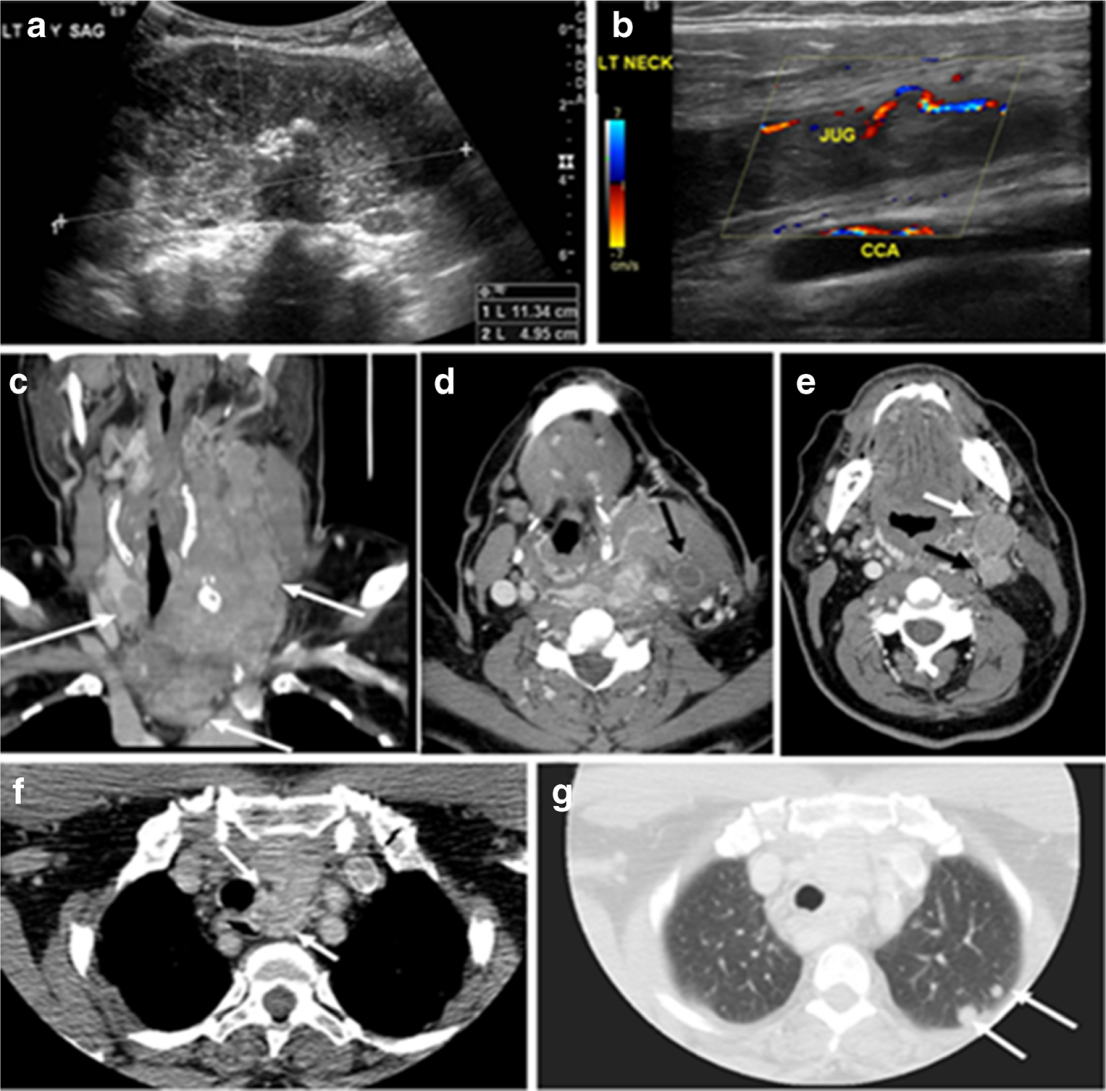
A poorly differentiated invasive left thyroid mass in a 58-year-old female patient. a Sagittal greyscale neck ultrasound shows a large hypoechoic lesion with macro-calcification and micro-calcification. b Sagittal colour Doppler ultrasound shows left internal jugular vein filling defect with detected internal vascularity suggestive of tumour thrombus. c Enhanced axial and coronal CT scans of the neck show heterogeneously enhancing large lesion replacing the left thyroid lobe and extending to the isthmus and the medial aspect of the right thyroid lobe (*white arrow*). The mass and the conglomerate lymph nodes measure 12.5 × 7 × 5.8 cm (*white arrows*). d, e Axial enhanced CT scans show enlarged left cervical nodes (white arrow) and left internal jugular vein (IJV) thrombus (black arrows). Note the IJV distention and central enhancing portion in the upper cut (*black arrow* in e) concerning the tumour thrombus. f, g Enhanced axial CT scan of the upper chest demonstrate a mass extension into the retrosternal area, left tracheoesophageal groove, and posterior to the trachea (*white arrows* in f). There are multiple bilateral pulmonary nodules (*white arrows* in g)
